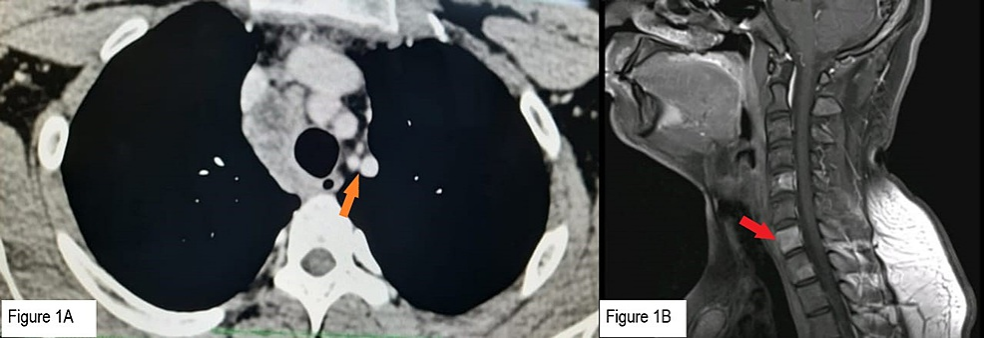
Radiological images before treatment. (A) Orange arrow: CECT thorax showing mediastinal lymphadenopathy. (B) Red arrow: CEMRI Spine showing contrast-enhancing lesions in vertebrae. CEMRI: contrast-enhanced magnetic resonance imaging, CECT: contrast-enhanced computed tomography.
